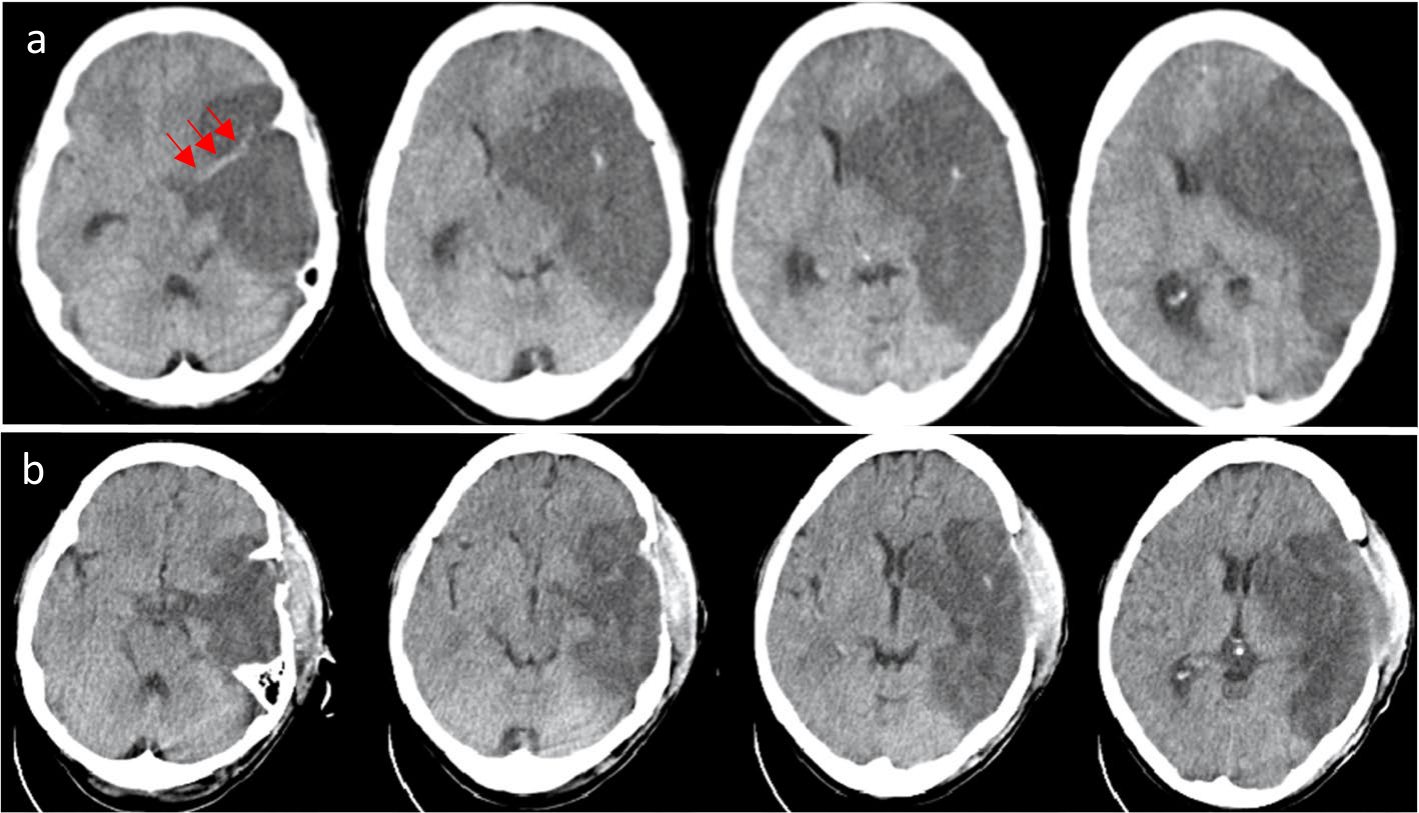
a Non-enhanced cranial computed tomography (NECCT) of a 44 gentleman with right side dense MCA sign (red arrows), mass effect and midline shift. b NECCT after surgery. Size of craniectomy is suboptimal. Surgery resulted in subdural hematoma but midline shift has resolved
